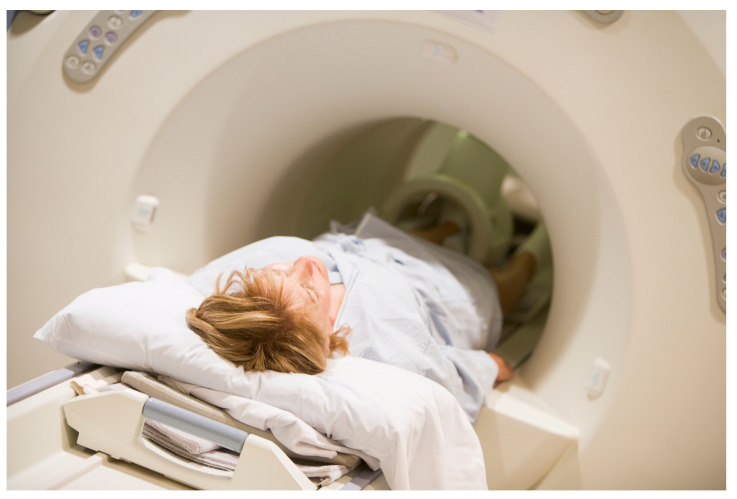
A CT examination of a patient to scan the abdominal cavity and liver (courtesy of @ monkeybusiness/Depositphotos.com).
